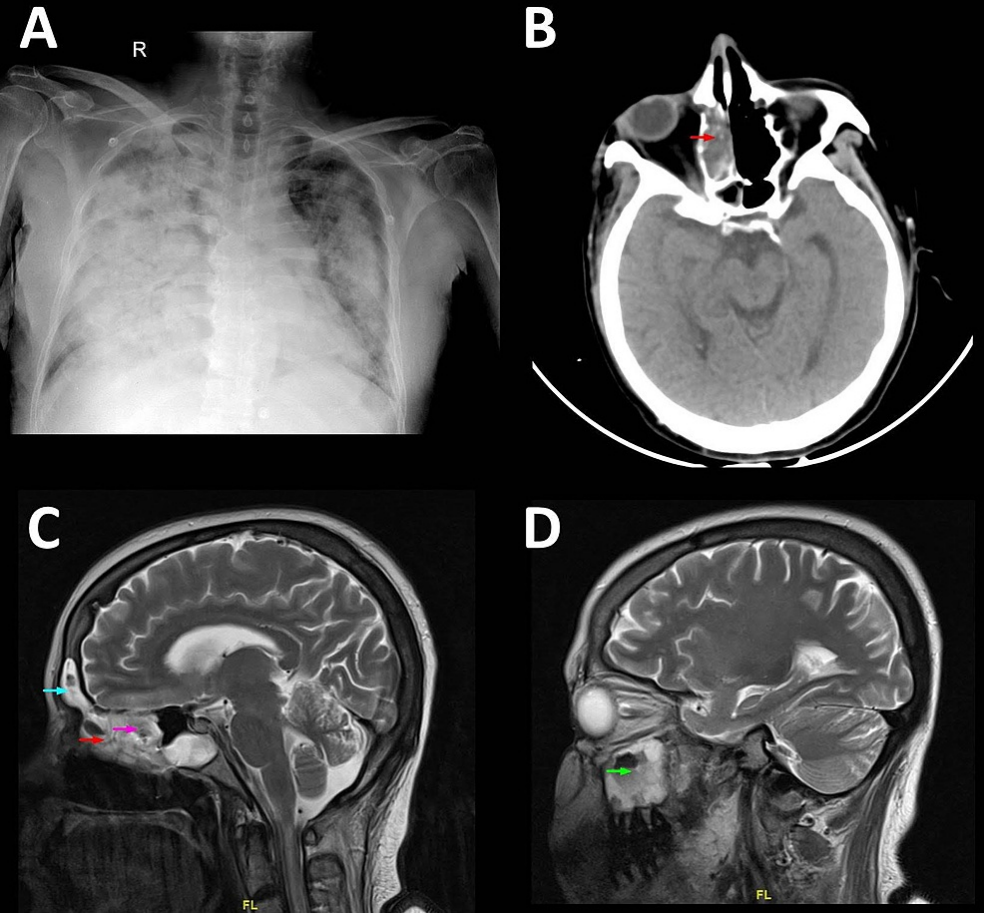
Representative images (A) Chest X-ray of a patient with mucormycosis; (B) axial non-contrast computed tomography of head showing right ethmoid sinusitis (red arrow); (C) T2 MRI head sagittal section showing right ethmoid sinusitis (red arrow), sphenoid sinusitis (magenta arrow) and right frontal sinusitis (blue arrow); (D) maxillary sinusitis (green arrow); the sinusitis is visualized as increased mucosal thickening suggesting fungal infection.
